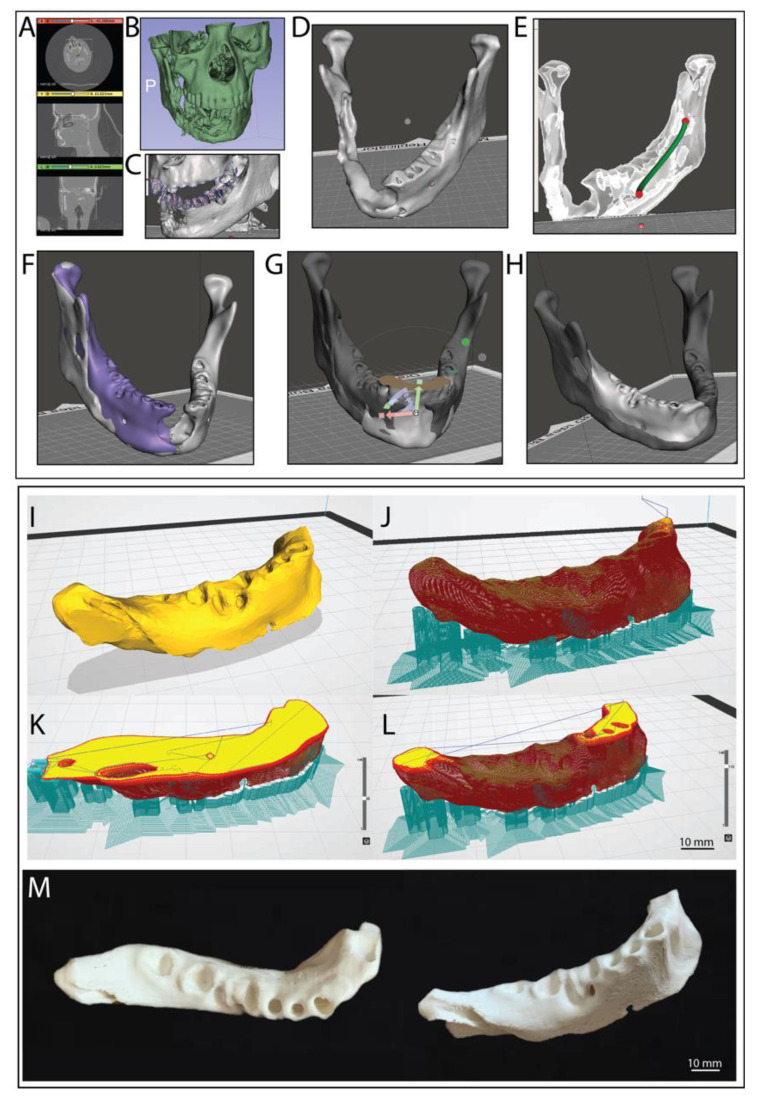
(A) CT scan of skull in the process of being cropped in order to isolate jaw; (B) segmented CT scan converted into a 3D model; (C) removing teeth and any joining surfaces between the skull and jaw; (D) Isolating jaw from skull via inverse election and converting the mesh to a solid; (E) Adding channel in order to preserve and define mandibular nerve channel; (F) Duplicating and mirroring of healthy side in order to replace missing jaw; (G) Filling gap in front using a CT scan of a healthy jaw; (H) Final implant after sculpting and smoothing; (I–L) Mandible Implant structure as seen in the Ender slicing software shown as different height regions, (M) 3D printed structure printed with a 0.2 mm nozzle at 215 °C.
